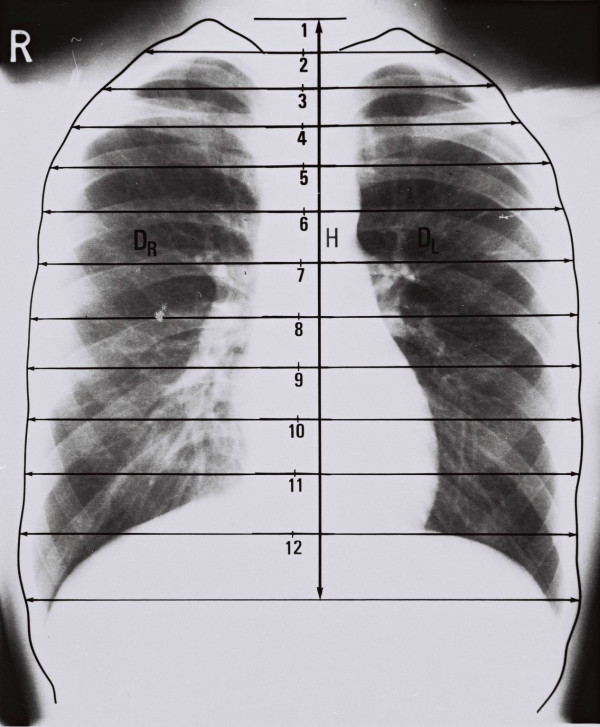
The method of thoracic ratios. Chest radiograph to show the method of measurement of calculation of thoracic ratios (TRs). H represents the distance from the upper end-plate of the T_1 _vertebral body and the lower end plate of T_12 _vertebral body. D_R _(D_L_) represents the distance from the midpoint of the distal end-plate of each vertebra body (T_1–12_) to the outline of the lateral border of the right (left) thoracic cage. These distances are standardised by dividing by the measured T_1 _– T_12 _distance (H). They are termed segmental right and left thoracic ratios TRs. Ratios are also calculated segmentally for the total width of the chest (right plus left measured lengths), [12].
